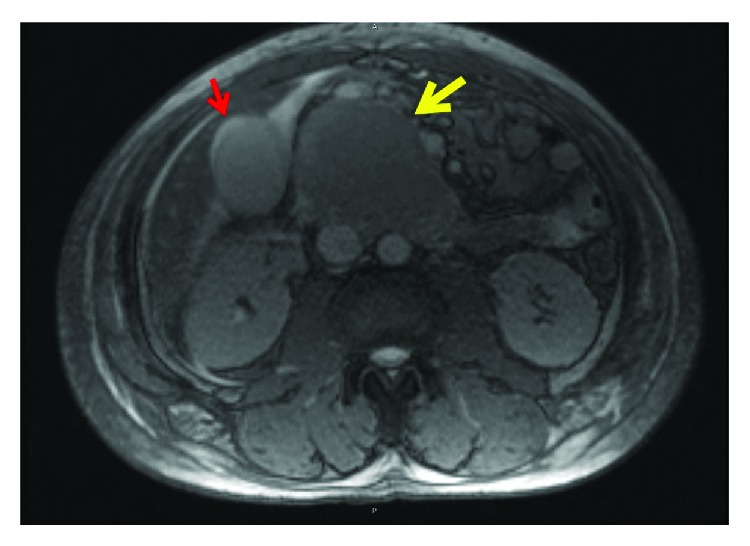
MRI of abdomen T2 sequence showed a moderately T2 hyperintense, round, well-circumscribed homogeneous mass, measuring approximately 6.3 × 5.9 × 8.2 cm, arising off the head of the pancreas (yellow arrow). There was also a mild thickening of gallbladder wall (red arrow).
